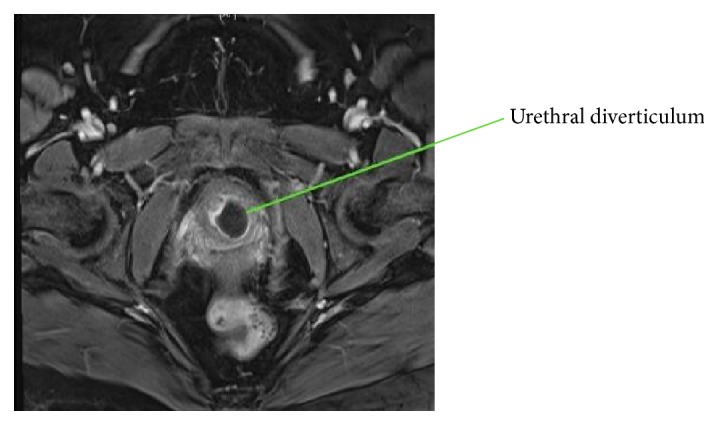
Pelvic MRI, axial view of urethral diverticulum.
